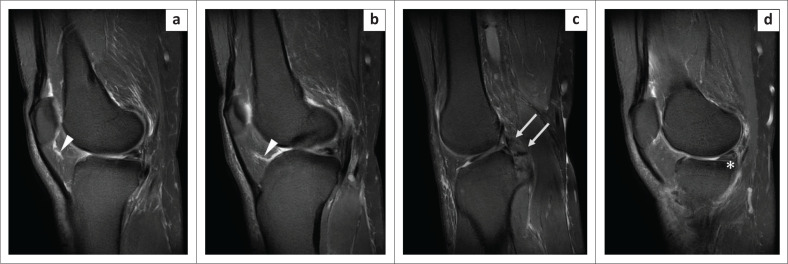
Magnetic resonance imaging of an infrapatellar plica tear in a 46-year-old male with a history of falling from a bike and knee pain: Serial oblique sagittal PDFS images (a) and (b) demonstrate a hyperintense fluid collection along the entire course of infrapatellar plica (arrow heads), with ragged margins. Oblique sagittal PDFS image (c) revealed that the patient also had an avulsion injury of the posterior cruciate ligament (arrows). Sagittal PDFS (d) indicated a complex tear of the posterior horn of the medial meniscus (asterisk).
